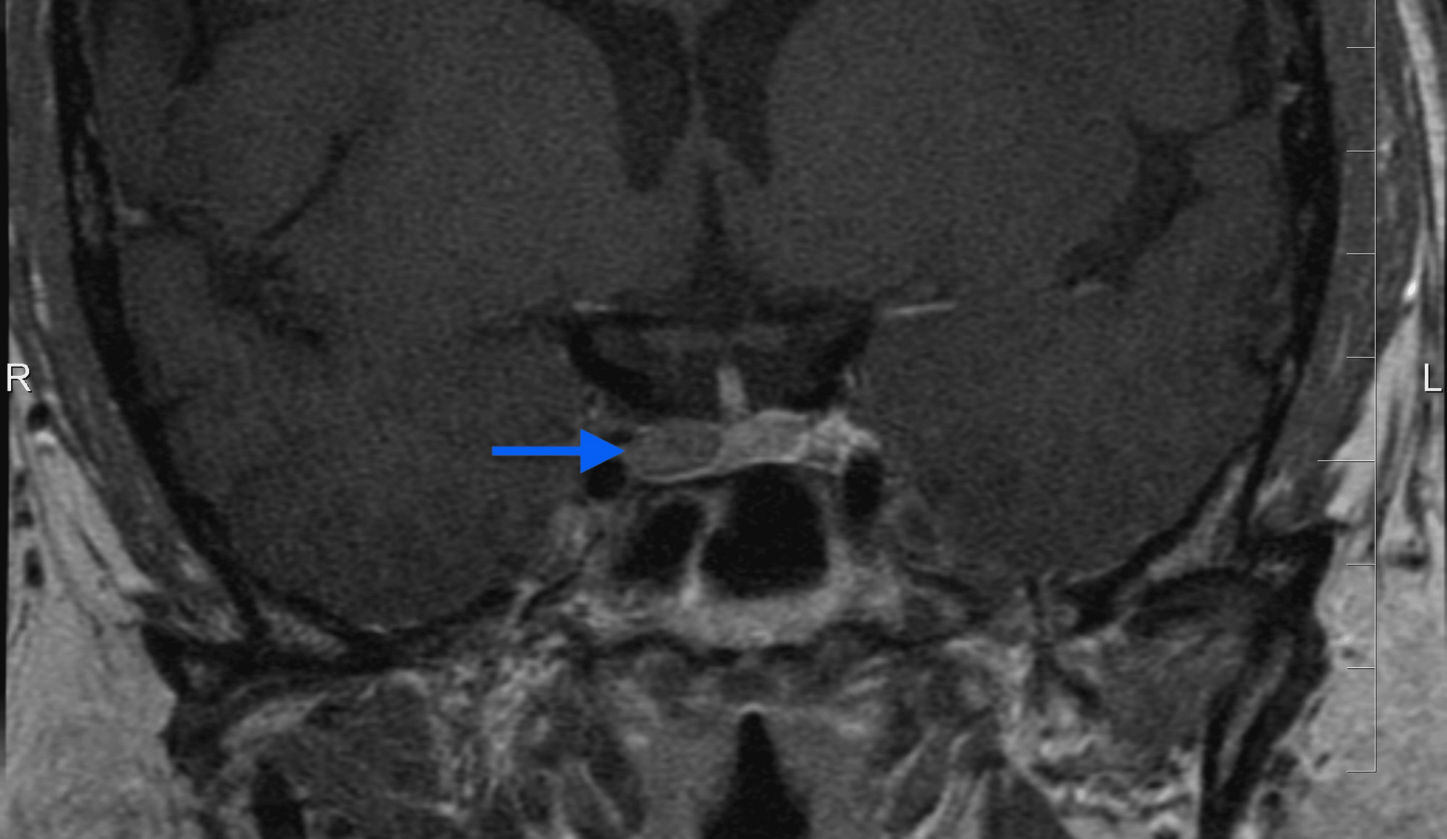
MRI evidence of pituitary adenoma recurrence (blue arrow)
